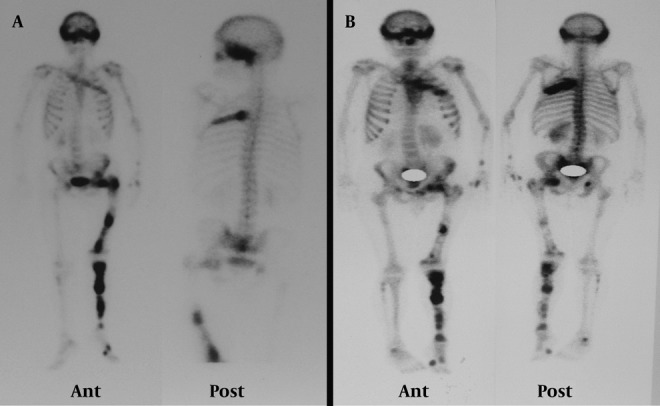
Patient’s previous whole body bone scan (A) showed increased osteoblastic activity in the cranium, left hemithorax, bilateral upper and lower extremities and pelvic bones. The recent bone scan (B) shows that the polyostotic FDB lesions are compatible with the previous bone scan and the recent FDG PET/CT scan.
